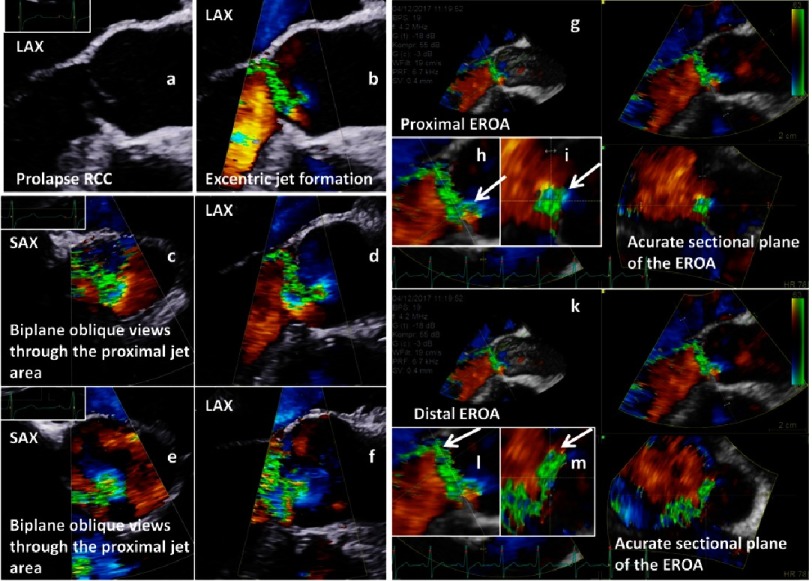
Assessment of the EROA by 2D-echocardiography (a-f) and 3D-echocardiography (g-m); simultaneous long axis view (LAX) of an AV prolapse during diastole (a - native; b - color-coded); color-coded biplane views of the regurgitant jet documenting oblique sectional planes of the regurgitant area in the short axis view (SAX) (c - SAX; d - LAX); second biplane color-coded approach to document the regurgitant jet area (e - SAX; f - LAX); 3D analysis of the entry of the regurgitant jet by post-processing of 3D data sets (g), longitudinal documentation of the central jet formation (h) and accurate perpendicular sectional plane for determination of proximal EROA (i); 3D analysis of the exit of the regurgitant jet by post-processing of 3D data set (k), longitudinal documentation of the central jet formation (l) and accurate perpendicular sectional plane for determination of distal EROA (m).
